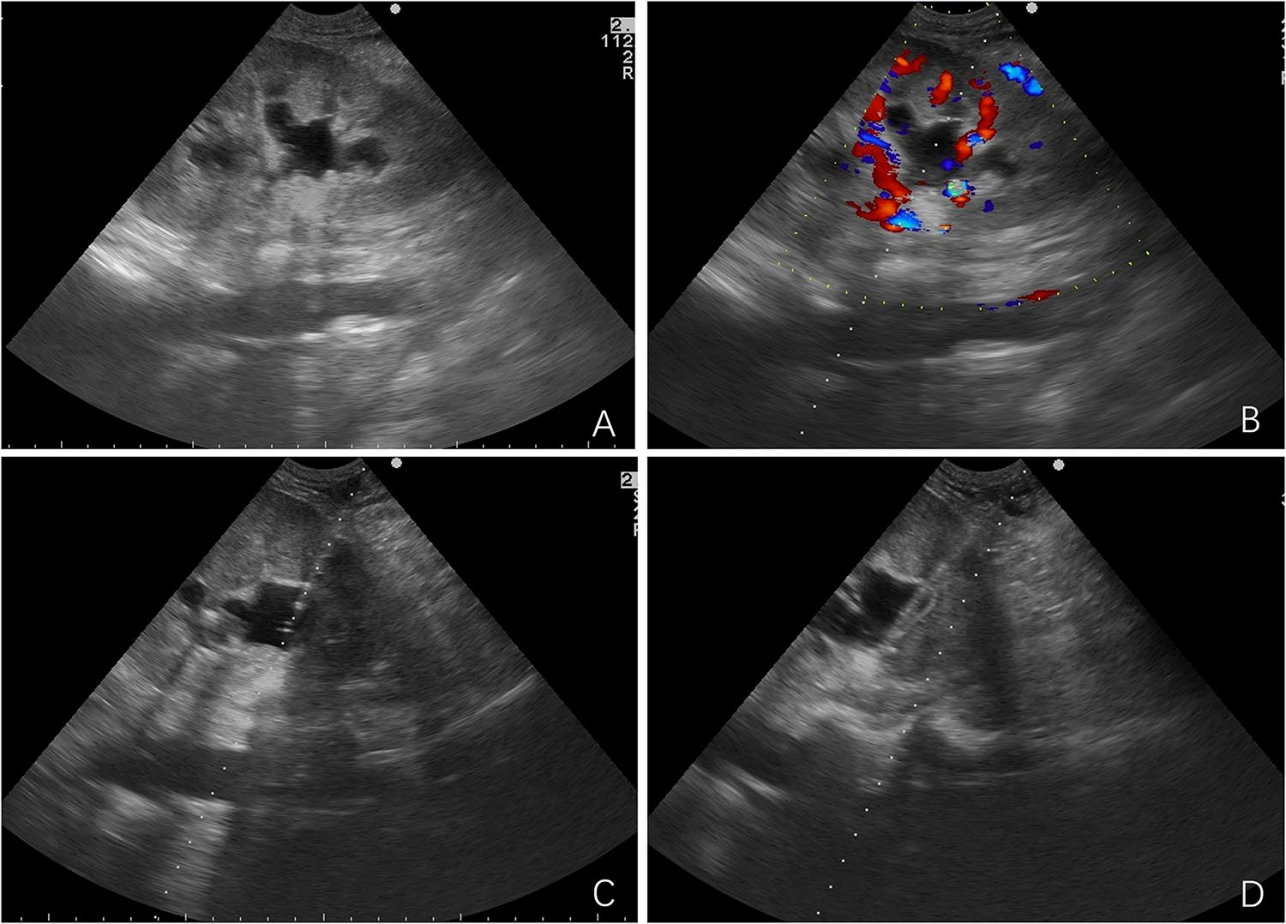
Method of ultrasound-guided percutaneous nephrostomy: A Gray-scale ultrasound showed hydronephrosis; B Color Doppler ultrasound was used to plan the puncture path; C Insertion of the drainage tube kit by puncture; D The drainage tube was placed in the renal pelvis
